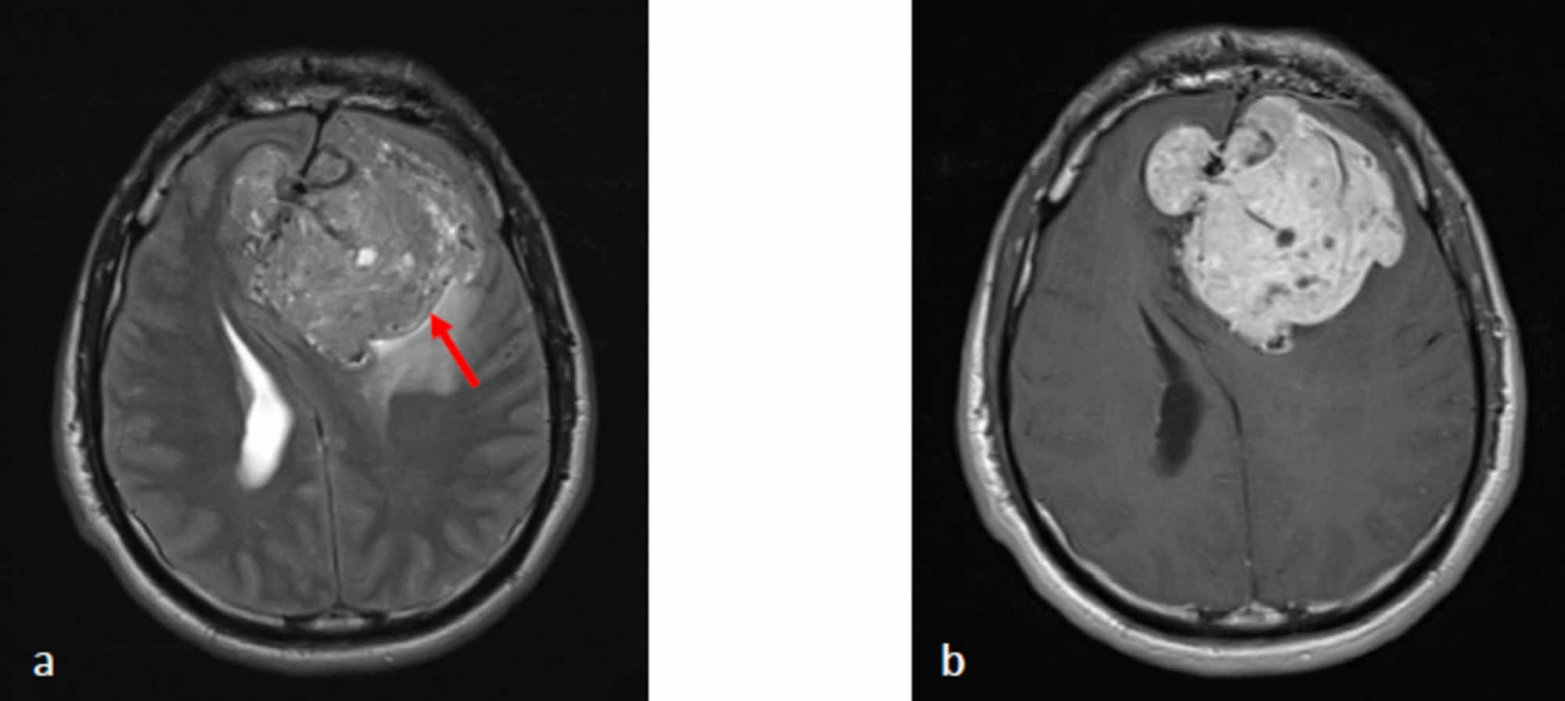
MRI (a) axial T2WI image shows the heterogeneous signal intensity of the mass, which is iso to mildly hypointense to the gray matter with cystic foci and multiple signal voids representing vessels. There is mild perilesional edema with significant mass effect and midline shift to the right. CSF cleft sign (red arrow) is seen denoting the extra-axial origin of the mass. (b) T1 post-contrast image shows an avid enhancement of the mass. MRI: magnetic resonance imaging; CSF: cerebrospinal fluid
